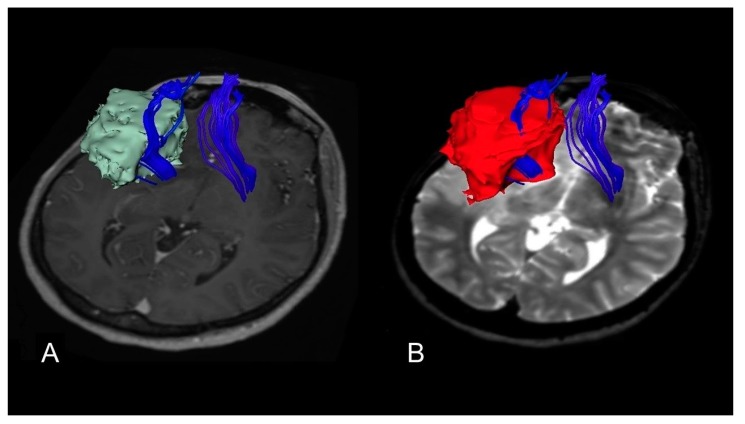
A case of insular DLGG infiltrating the Cortico-Spinal tract. The preoperative tumor volume computed on post-contrast T1-weighted magnetic resonance imaging (MRI) was 58 cm^3^ (axial slices (A)). The preoperative tumor volume computed on T2-weighted MRI was 83 cm^3^ (axial slices (B)). The preoperative ΔT2T1 MRI index was 25 cm^3^. The prevalence of the diffusive and infiltrative growth generates the tumor diffusion along the white matter, resulting in a complex shape with digitations more visible on T2-weighted images. The patient was in Engel Class IB 18 months after surgery.
